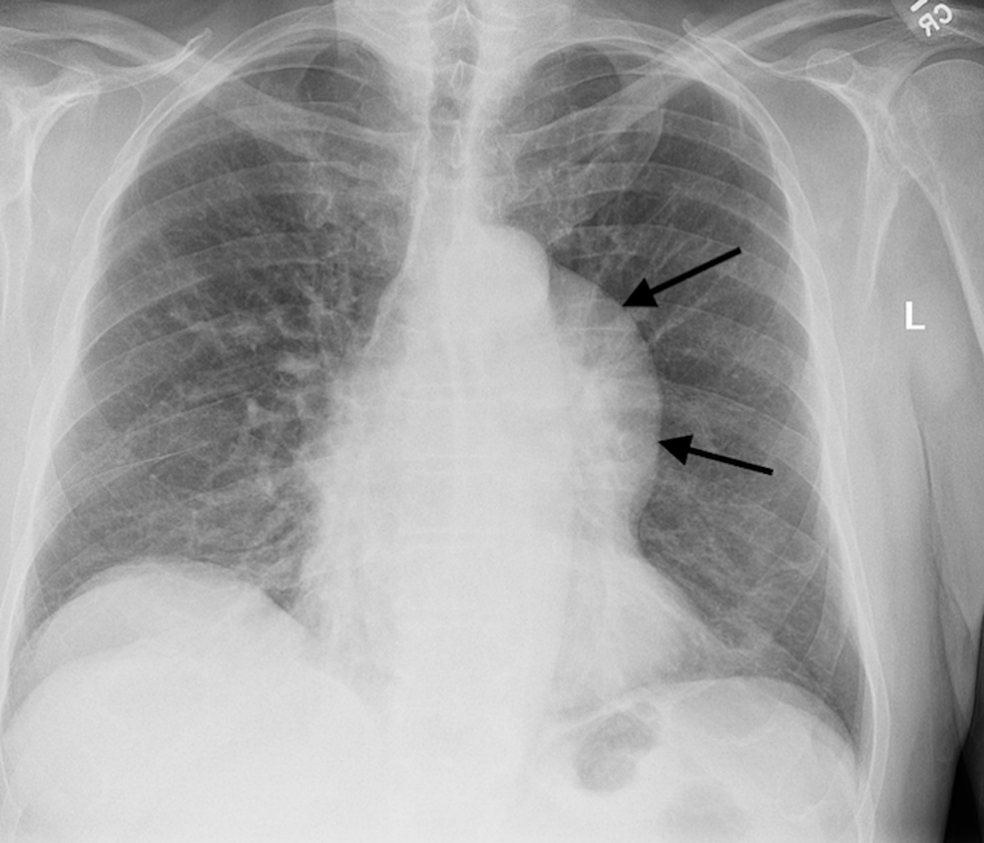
Posteroanterior (PA) chest radiograph of the anterior mediastinal mass (as indicated by the arrows). It is identified by the hilum overlay and preservation of posterior mediastinal lines.
